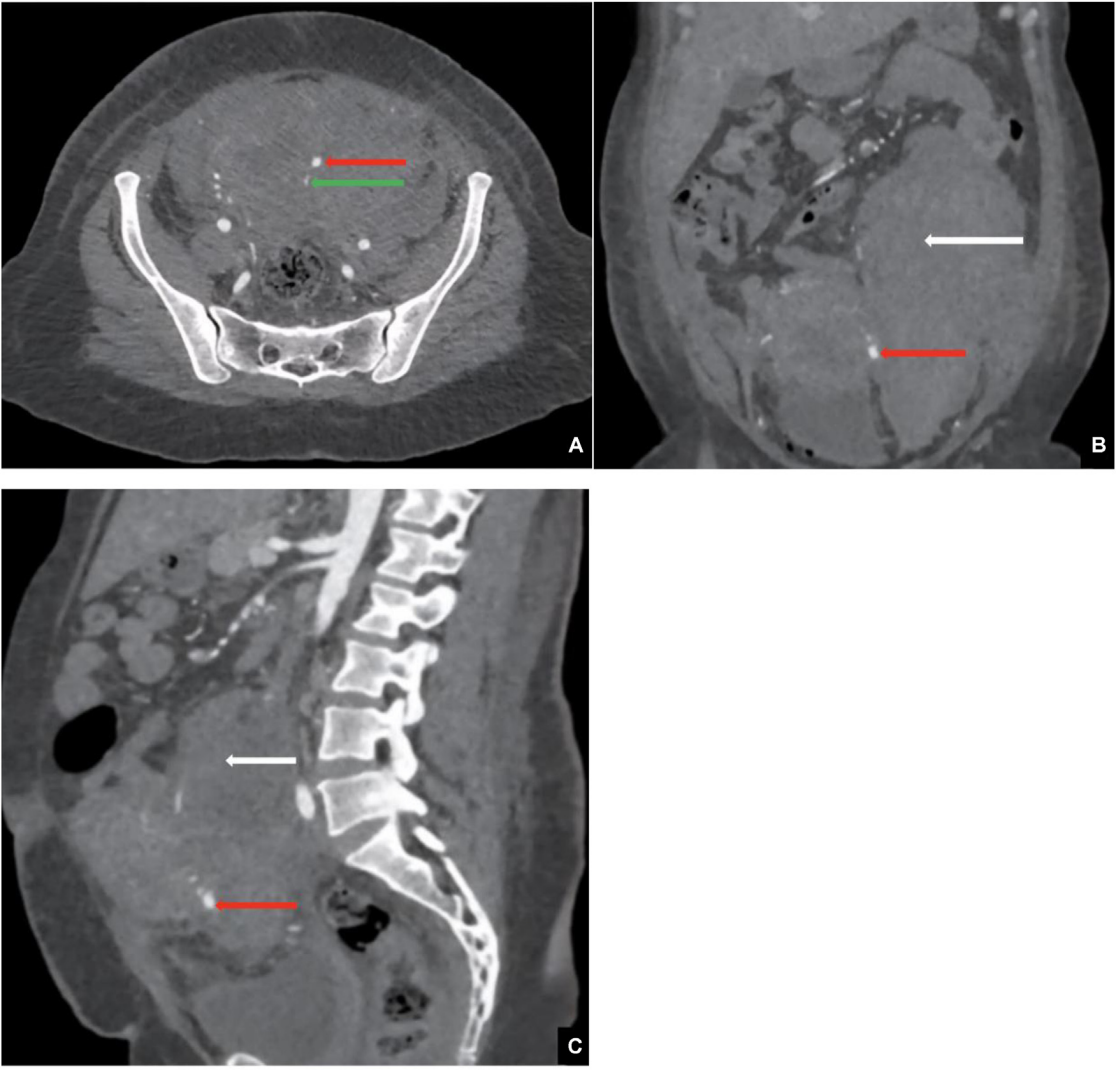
Arterial phase contrast-enhanced CT image (A) axial, (B) coronal and (C) sagittal: showing a well-defined pseudoaneurysm (red arrow) arising from the left uterine artery (green arrow), associated with a retroperitoneal hematoma (white arrow).
